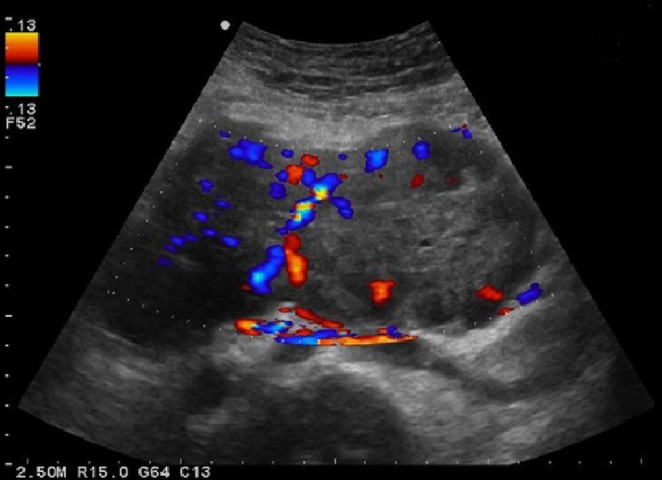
Ultrsound Doppler demonstrating hypervascularity of the mesenteric 15 × 7.2 × 13 cm mass.
